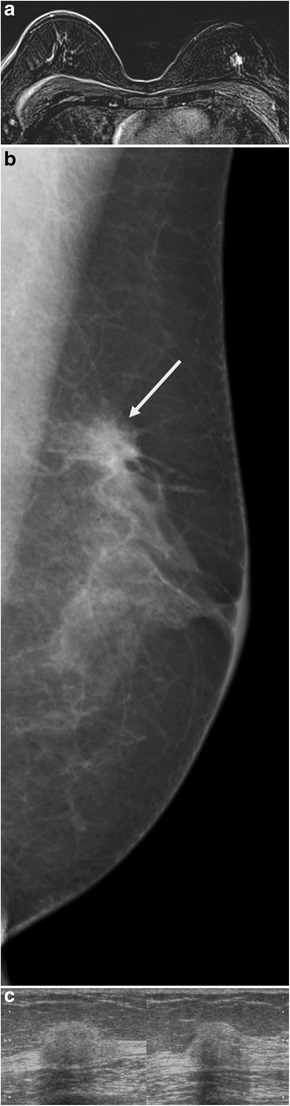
Invasive ductal carcinoma. Annual surveillance in a patient at very high risk already treated for left breast cancer. a MRI, gadolinium-enhanced T1 subtraction sequence, demonstrating an irregular, poorly circumscribed mass at the junction of the upper quadrants of the left breast. The gadolinium-enhanced examination shows initial 90% enhancement with washout at the late phase. b Mammography guided by MRI in the same patient, oblique view: demonstration of a non-calcified mass (*arrows*), with architectural distortion at the junction of the upper quadrants of the left breast. c MRI-guided ultrasound of the left breast in the same patient. At the junction of the upper quadrants, at the 12.30 position, 4 cm from the nipple, presence of a hyperechoic nodule with microlobular margins, with posterior attenuation classified as high ACR4. Microbiopsy demonstrated poorly differentiated invasive ductal carcinoma (grade II, low MI, HR+, HER2-)
